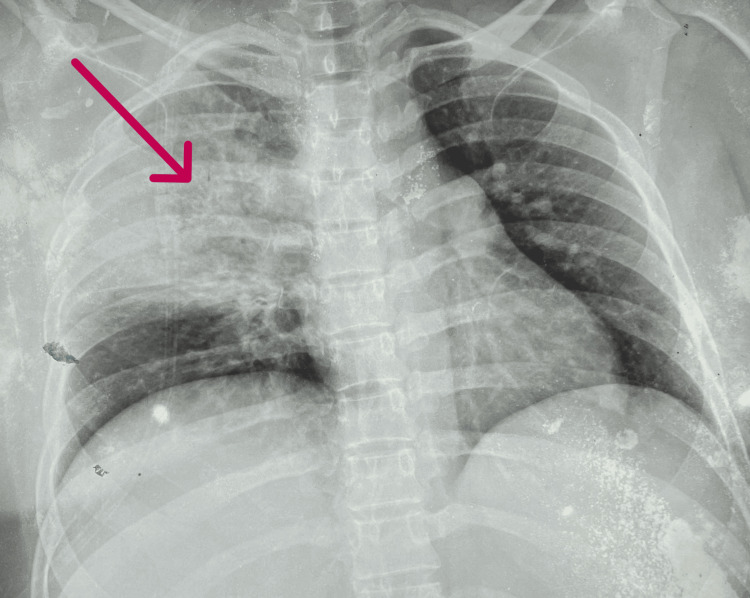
Chest X-ray on admission showing right-sided consolidation (arrow)
